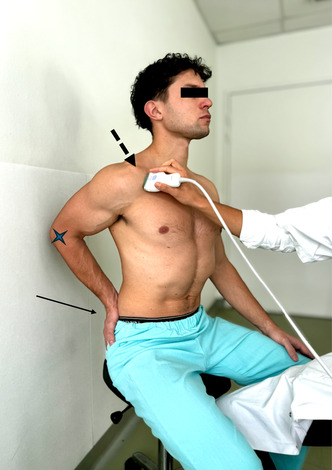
Ultrasound probe position. The patient places the hand on the lower back (arrow), elbow flexed at 90° and aligned with the trunck (blue star), in a modified Crass position. The probe is positioned in the deltopectoral groove to visualize the tendon along its full axis (dashed black arrow) in a coronal oblique plane.
